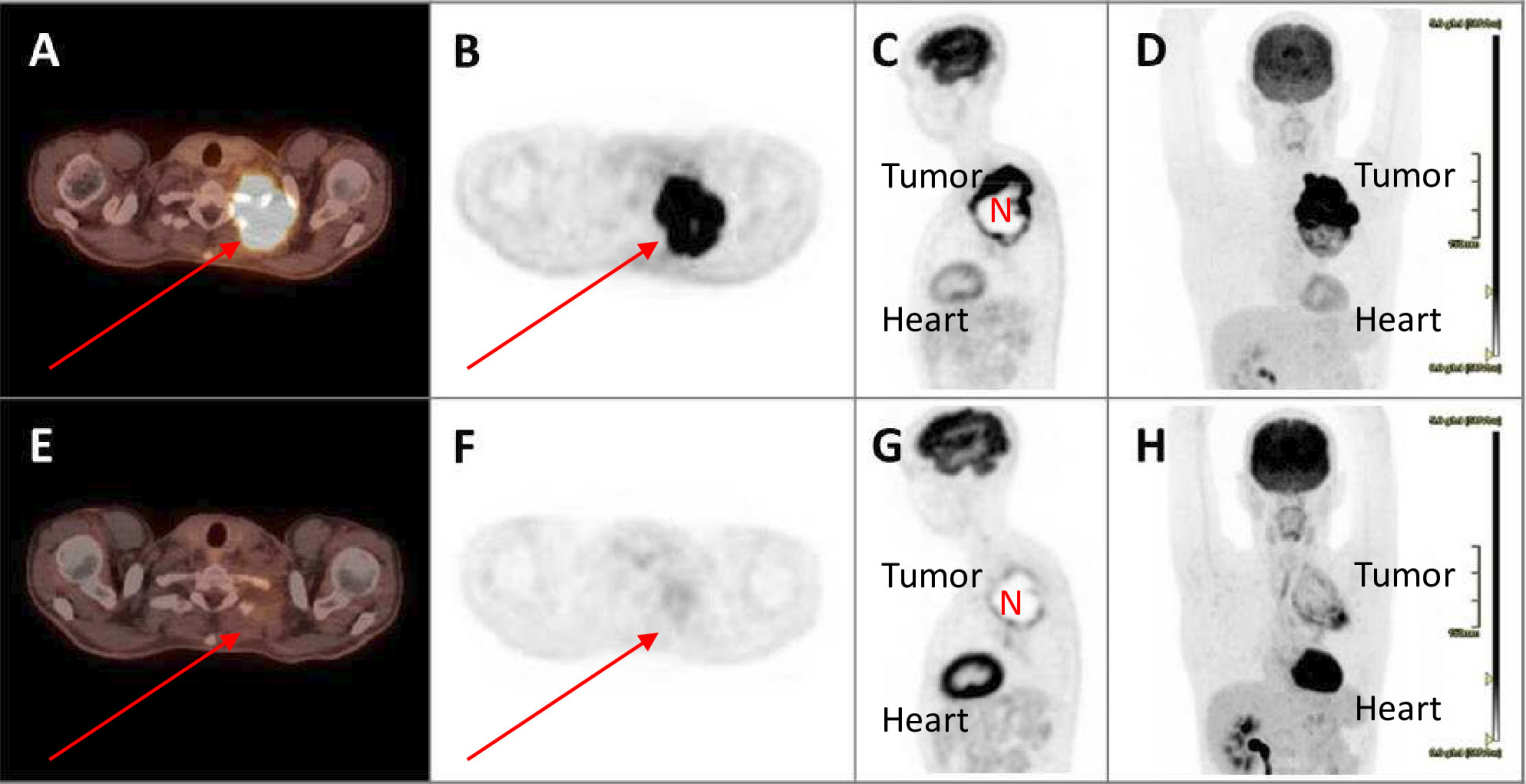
The FDG PET/CT images of an adult patient (23), before (top row) and after (bottom row) induction chemo-immuno-radiotherapy are shown, using fused PET/CT images (A,E) and CT-attenuation corrected PET images of axial (B,F), sagittal (C,G) and coronal view (D,H) reconstructions. This patient had a tumor in the left upper lobe with, at baseline, ingrowth in the thoracic wall and adjacent structures such as the musculature and left ribs (red arrows). The tumor had a necrotic center (N), and there was no apparent mediastinal lymph node involvement. CTAC, CT-attenuation correction; FDG, fluorodeoxyglucose; PET, positron emission tomography; RECIST, Response Evaluation Criteria in Solid Tumors.
